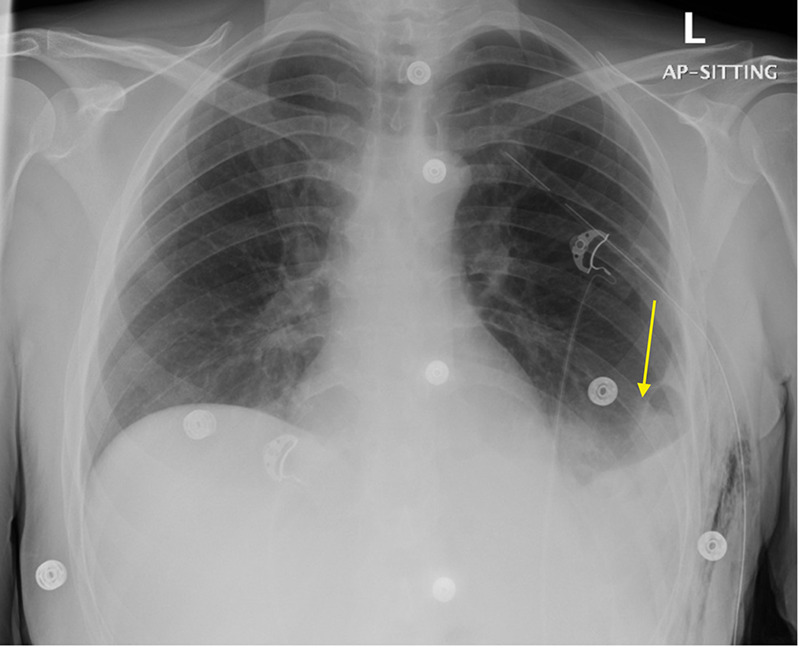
Postoperative chest x-ray, AP view. Showing reduced previously herniated abdominal organ (arrow).
